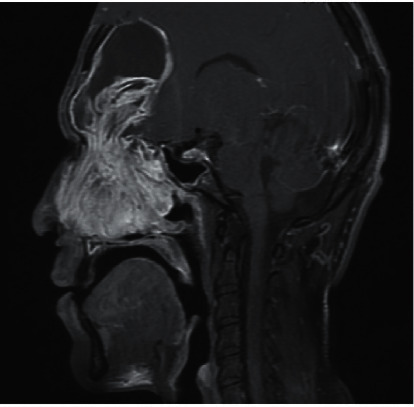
MRI of the brain and sinuses in the sagittal plane showing a significant expansion of both frontal sinuses caused by the mucopyoceles.
